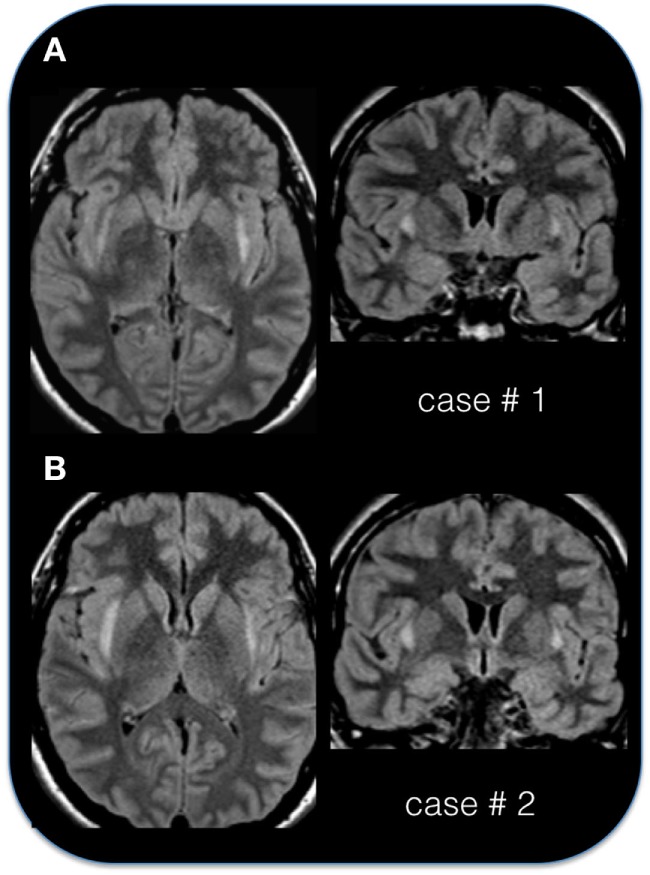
The “claustrum sign” in two patients. (A) Axial and coronal FLAIR sequence in case # 1, 29-year-old female, acquired 10 days after status epilepticus (SE) onset. (B) Axial and coronal FLAIR sequence in case # 2, 24-year-old female, acquired 4 days after SE onset. Note the hyperintense appearance of the capsula esterna/claustrum region in the two cases.
